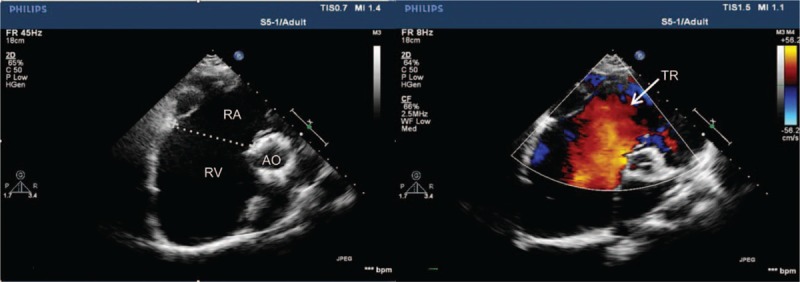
Preoperative echocardiography showing enlargement of the right chamber and severe tricuspid regurgitation. These images come from Case 1 and are representative of all 3 cases in this series. RA = right atrium, RV = right ventricle, TR = tricuspid regurgitation.
